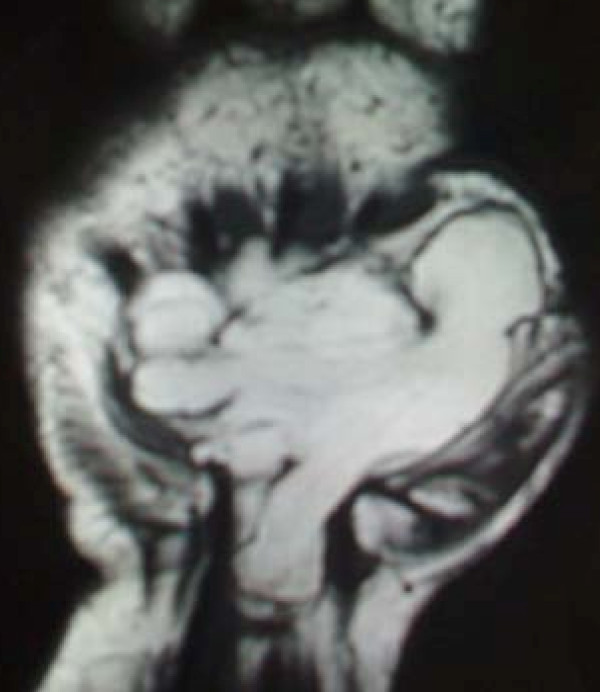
MRI picture showing one of the recesses invading the carpal tunnel.
